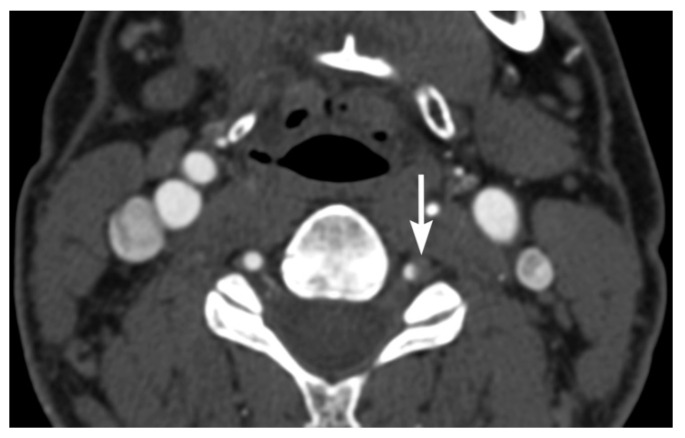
42-year-old male with left vertebral artery injury. Axial image multidetector CT angiographic image shows eccentric left vertebral artery intramural hematoma causing moderate eccentric narrowing of the arterial lumen.
